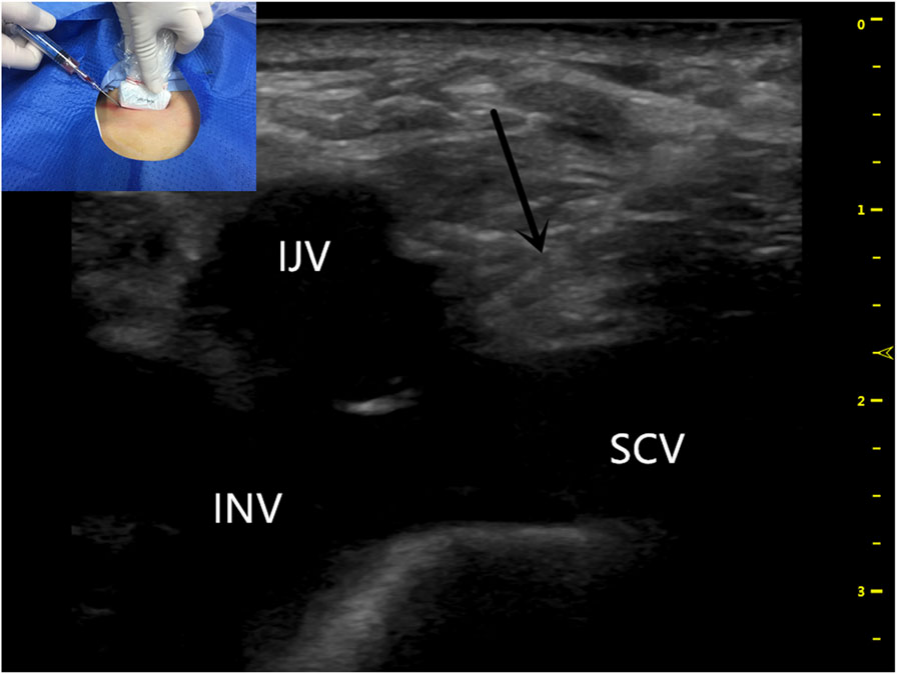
Ultrasound-guided successful puncture of right INV with an inserted needle (black arrow). INV longitudinal view, in-plane approach. INV indicates innominate vein. IJV indicates internal jugular vein. SCV indicates subclavian vein
